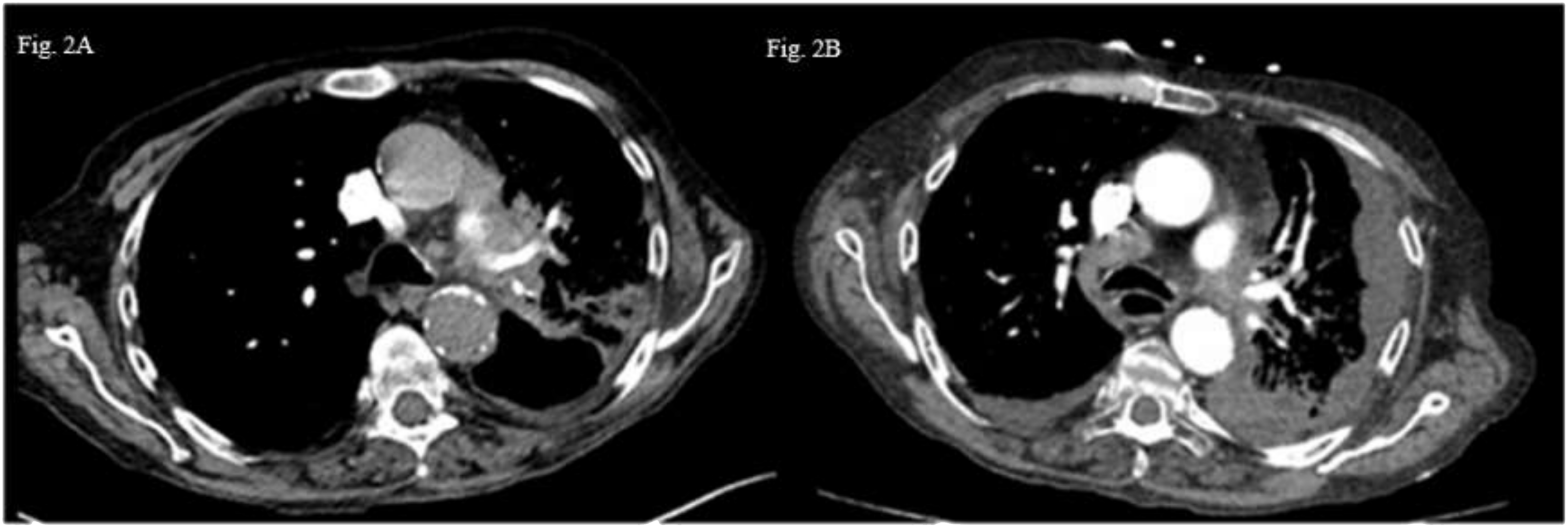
Thoraric CT-scan images axial section (A) Left hilar tumoral lesion. (B) Appearance of a left plural effusion and progression lower right paratracheal area lymph nodw (4R).
